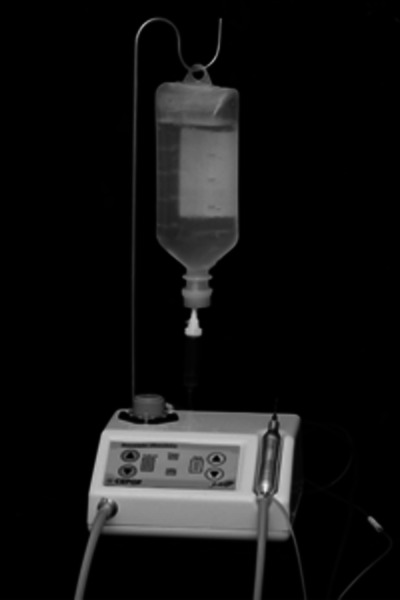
Ultrasound caries removal device.
